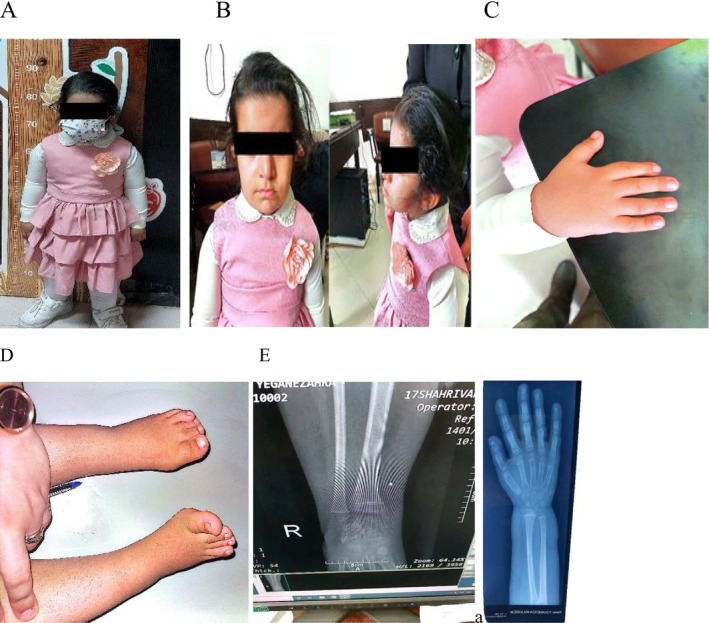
(A) Full‐body photograph at age 5, demonstrating disproportionate short stature (height: 88 cm, *Z* score −4.4; upper‐to‐lower body segment ratio: 1.8). (B) Clinical image highlighting facial dysmorphism, including frontal bossing, mild hypertropia of the right eye, low‐set ears, and a short neck, consistent with IMAGe syndrome. (C) Photograph of the right hand showing a shortened index finger. (D) Image of the feet demonstrating bilateral clinodactyly of the fourth toes, bending toward the third toes. (E) Radiographs of wrists and hips revealing osteonecrosis, metaphyseal dysplasia, and frayed bone edges.
